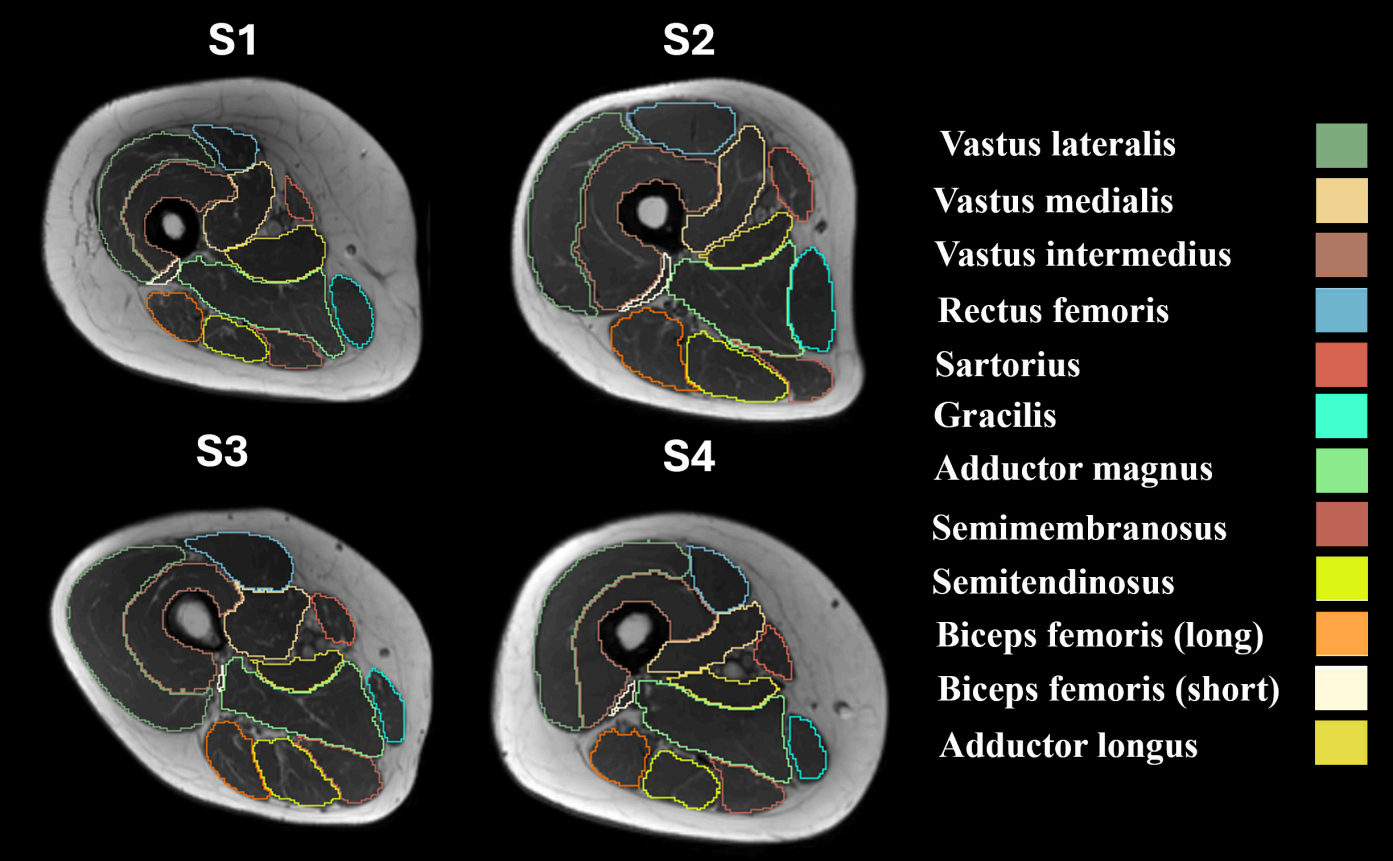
Representative axial magnetic resonance imaging cross-sections of the thigh for 4 participants (S1 to S4), with segmented anatomical regions representing individual muscles. Each muscle is outlined in a specific color corresponding to the legend on the right. Muscles include the vastus lateralis, vastus medialis, vastus intermedius, rectus femoris, sartorius, gracilis, adductor magnus, semimembranosus, semitendinosus, biceps femoris (long and short heads), and adductor longus.
